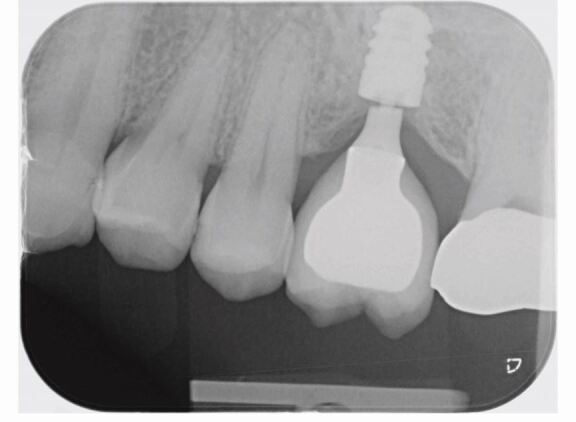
Periapical radiograph showing how a fractured abutment presents once the screw is accessed and undone
